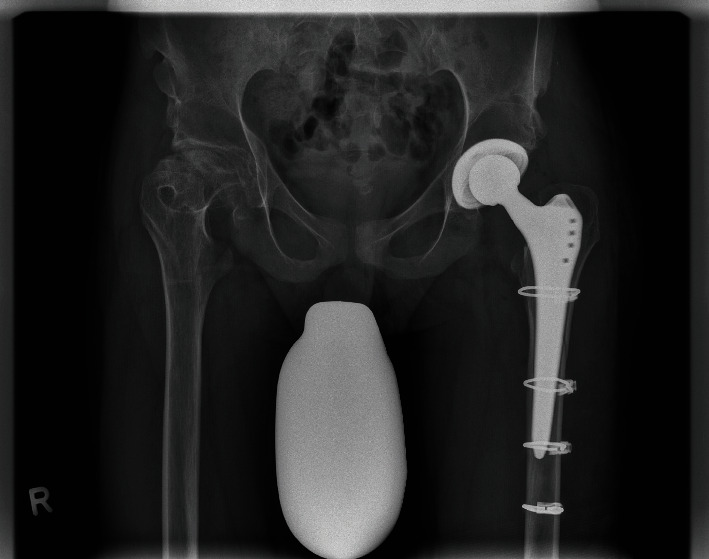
Osteosynthesis of the periprosthetic fracture. X-ray on the day of hospital discharge. The anteroposterior pelvic projections show the THA and osteosyntheses. The fracture has been reduced and fixed with four cable cerclages. The patient was restricted to 20 kg weight-bearing on the left leg for 6 weeks.
